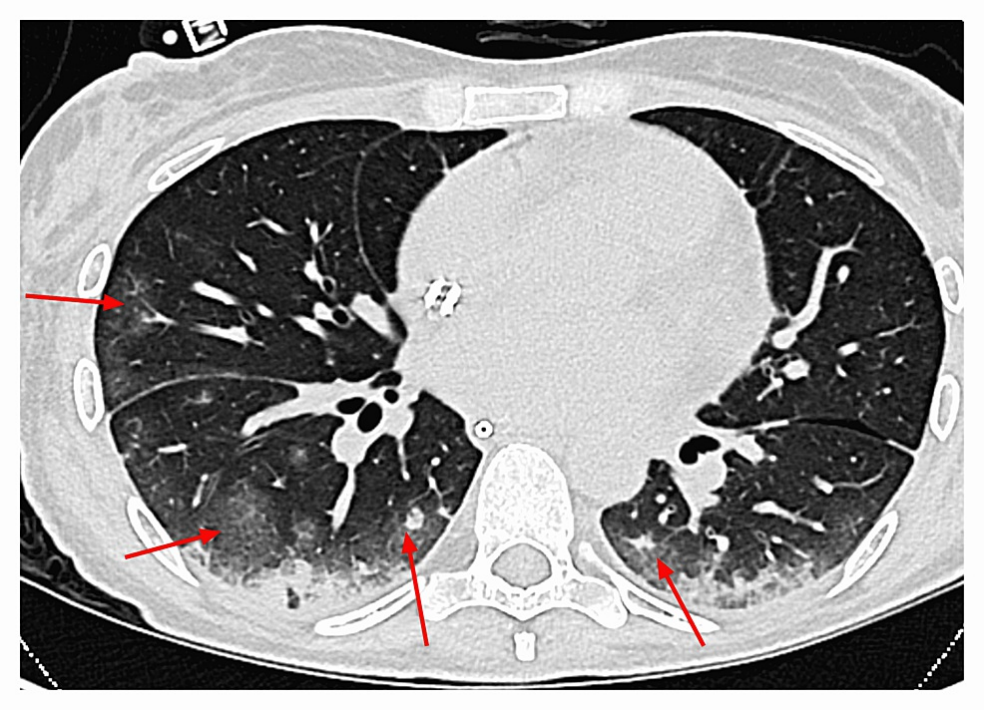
Computed tomography scan of the chest showing bilateral, multilobar, peribronchial consolidations with ground-glass opacities.
